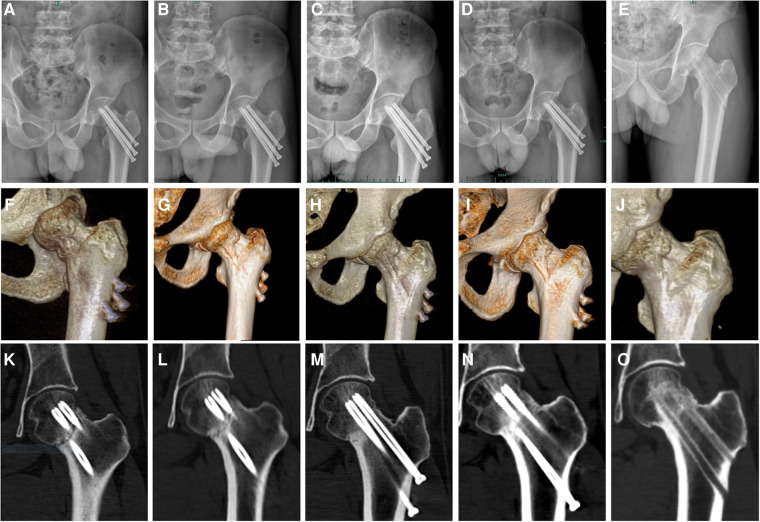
Radiological images (A, F, K) showing trabeculae bridging through fracture sites and a reduction of the fracture space after 3 months of taking the drug. Representative images of the patient regularly reviewed while taking medication (B, G, L). The x-ray (C) and CT film (H, M) showing the bone healing completely after 6 months of stopping the drug. Representative images of the patient regularly reviewed after drug withdrawal (D, I, N). Internal fixation screws were removed in July 2021 (E, J, O) when the patient returned to normal activity feeling no pain.
